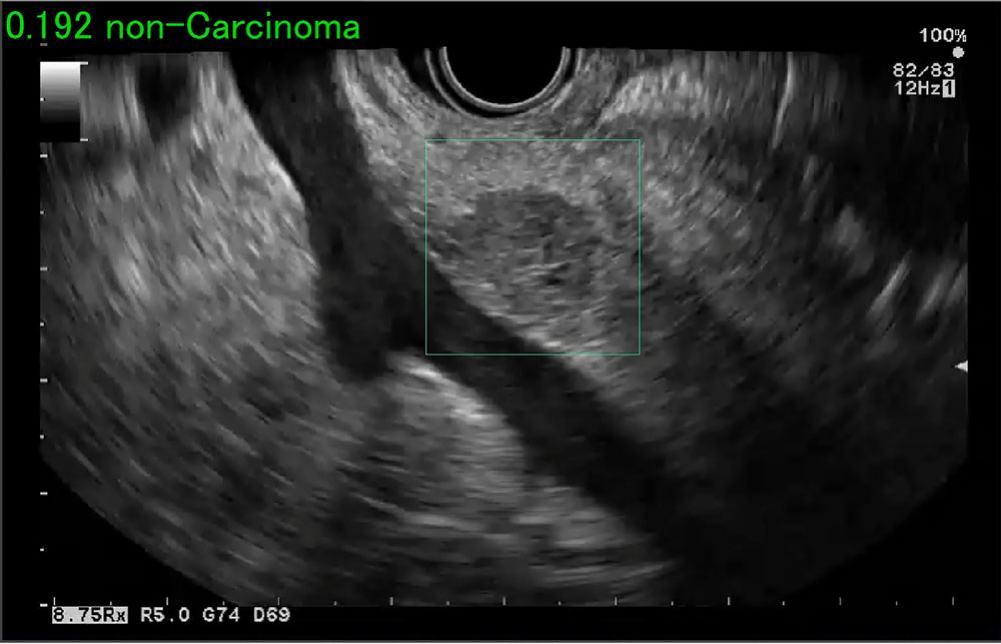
Artificial intelligence image for differential diagnosis of pancreatic masses. Endoscopic ultrasonography image (pseudo papillary neoplasms) is used for the diagnosis of carcinoma by artificial intelligence. The probability of one endoscopic ultrasonography image is expressed in the upper left, and AI diagnoses this lesion as non‐carcinoma.
